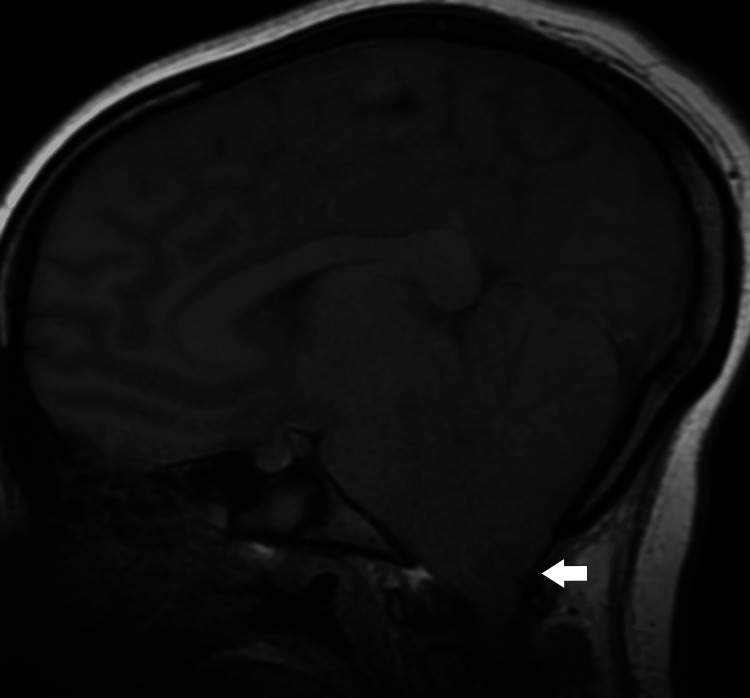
Magnetic resonance sagittal (T1/FLAIR) image on day three of admission showing cerebellar tonsillar herniation in brain (arrow) FLAIR: fluid-attenuated inversion recovery
